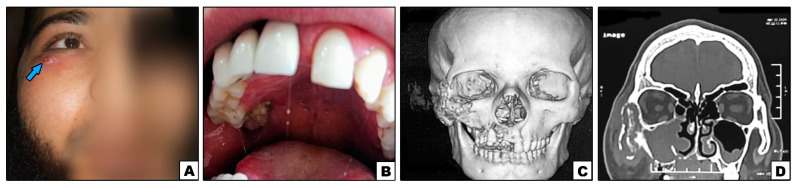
Case#2: (A) Extraoral swelling of the right side of the face (note: sinus tract inferolaterally to the eye {arrow}). (B) Exposed necrotic bone in the right posterior palate. (C,D) 3D and cronal CT showing bone destruction of right maxilla, inferior orbital rim, and lower part of zygoma.
